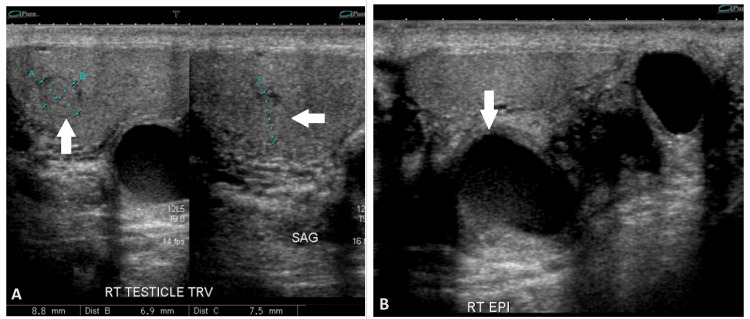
Ultrasound images of the right testicle A: Coronal and sagittal ultrasound view of the right testicle demonstrating a 9mm hypoechoic right testicular lesion as measured above. B: 2cm right-sided spermatocele within the epididymis.
